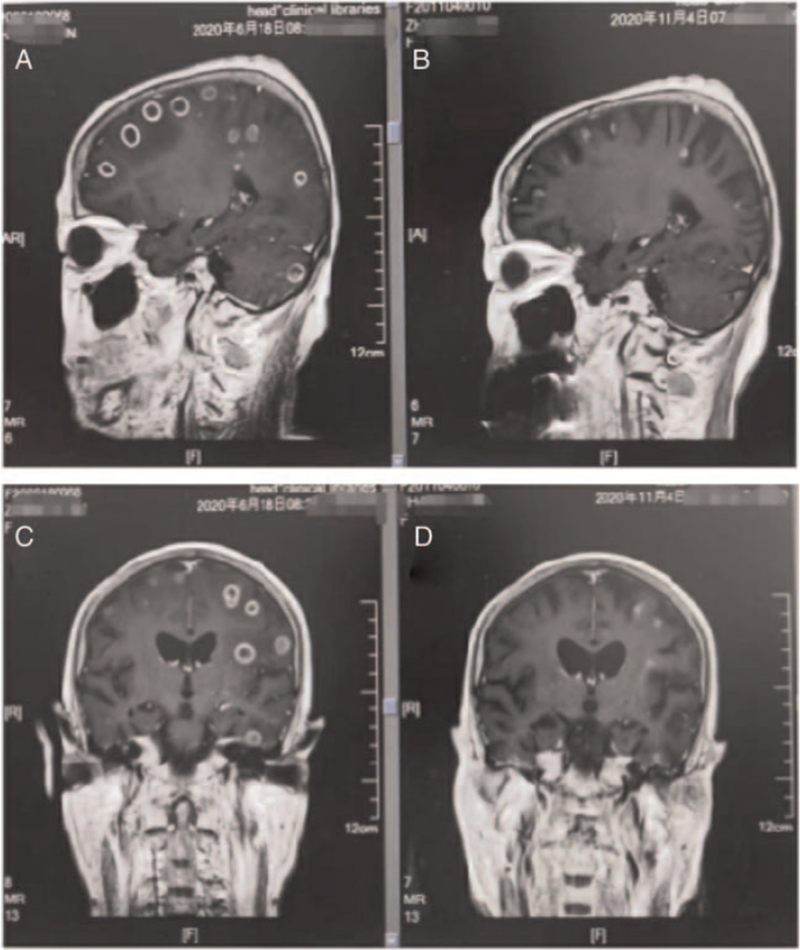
The brain magnetic resonance imaging (MRI) of different time showed multiple brain metastasis. (A,C) (2020-06-18) before “osimertinib” treatment. (B,D) (2020-11-04) after “osimertinib” treatment was administered for 4 months.
